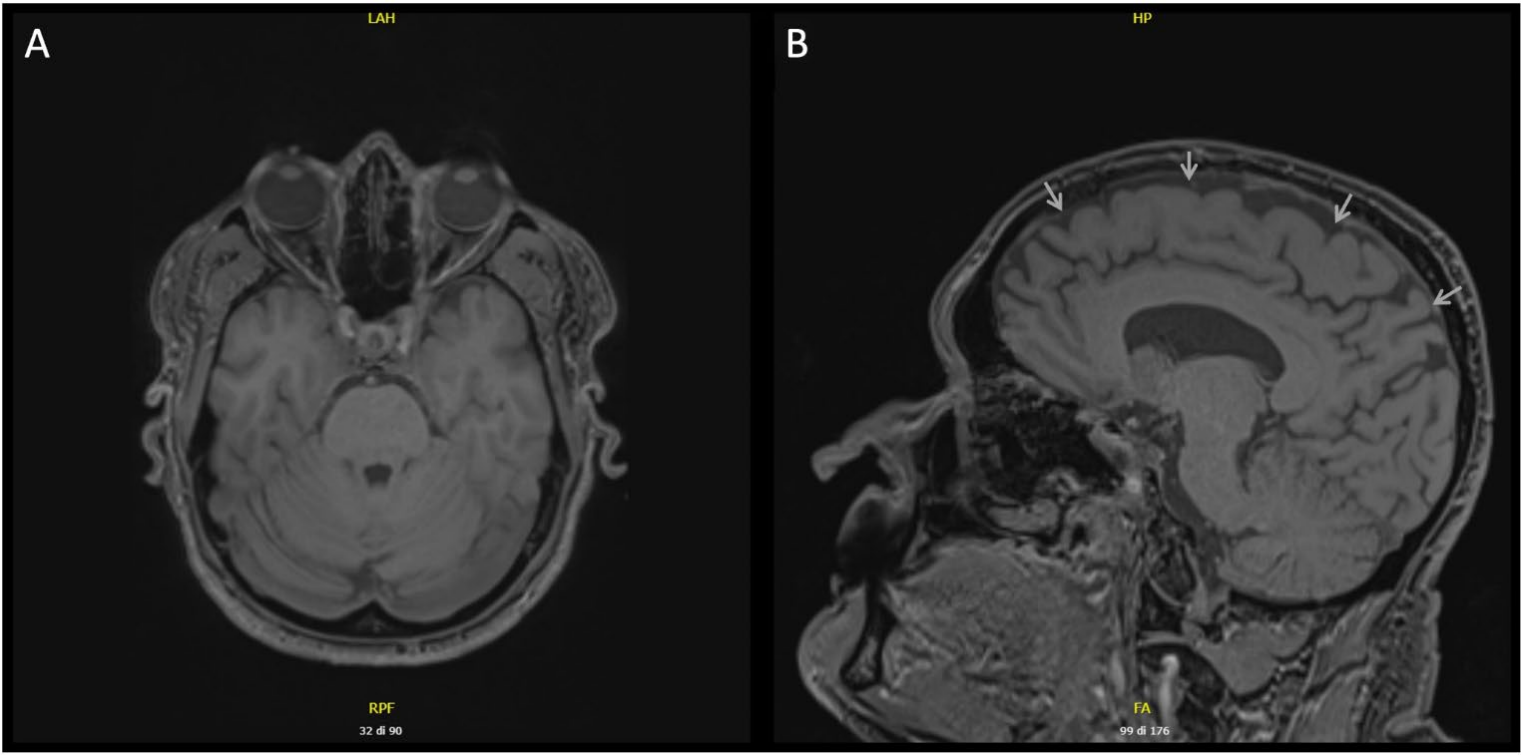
Brain magnetic resonance imaging (MRI): (A) T1-weighted axial image and (B) T1-weighted sagittal image show a slight widening of the subarachnoid spaces of the cranial vault (grey arrows) without evidence of cerebellar or brainstem atrophy
